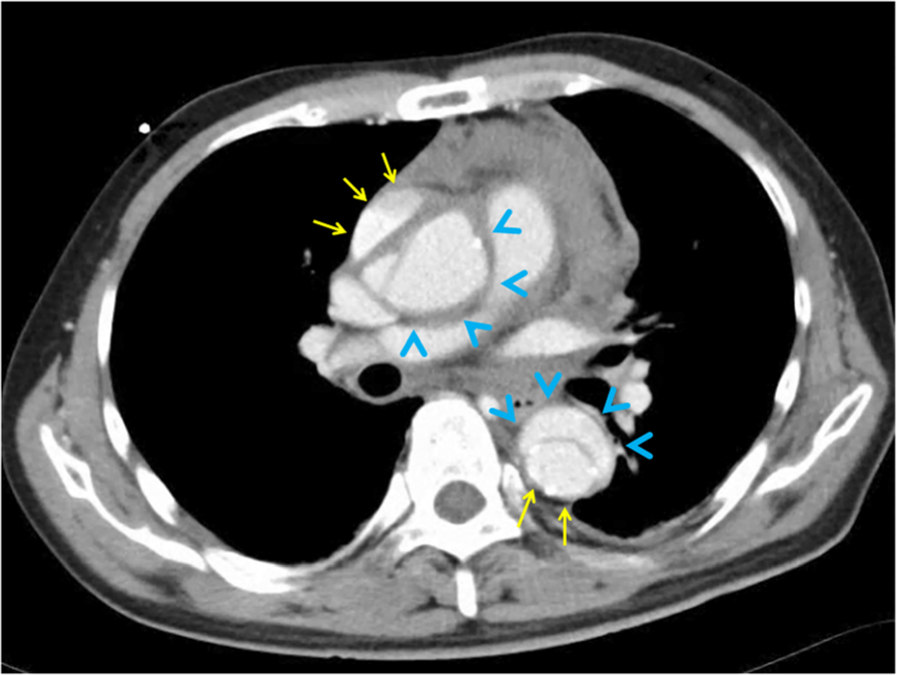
Computed tomography with contrast enhancement in a patient with acute aortic dissection. Arrows indicate the true lumen and arrowheads indicate the false lumen
